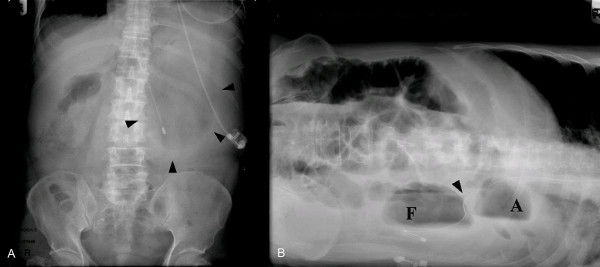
(A) Supine abdominal radiograph showing a dilated spherical gastric shadow. (B) Right lateral decubitus abdominal radiograph showing a double gastric bubble, with the superior bubble representing the antrum (A) and inferior bubble being the fundus (F). Nasogastric tube indentation at the cardio-oesophageal junction (arrow) as it enters the stomach.
